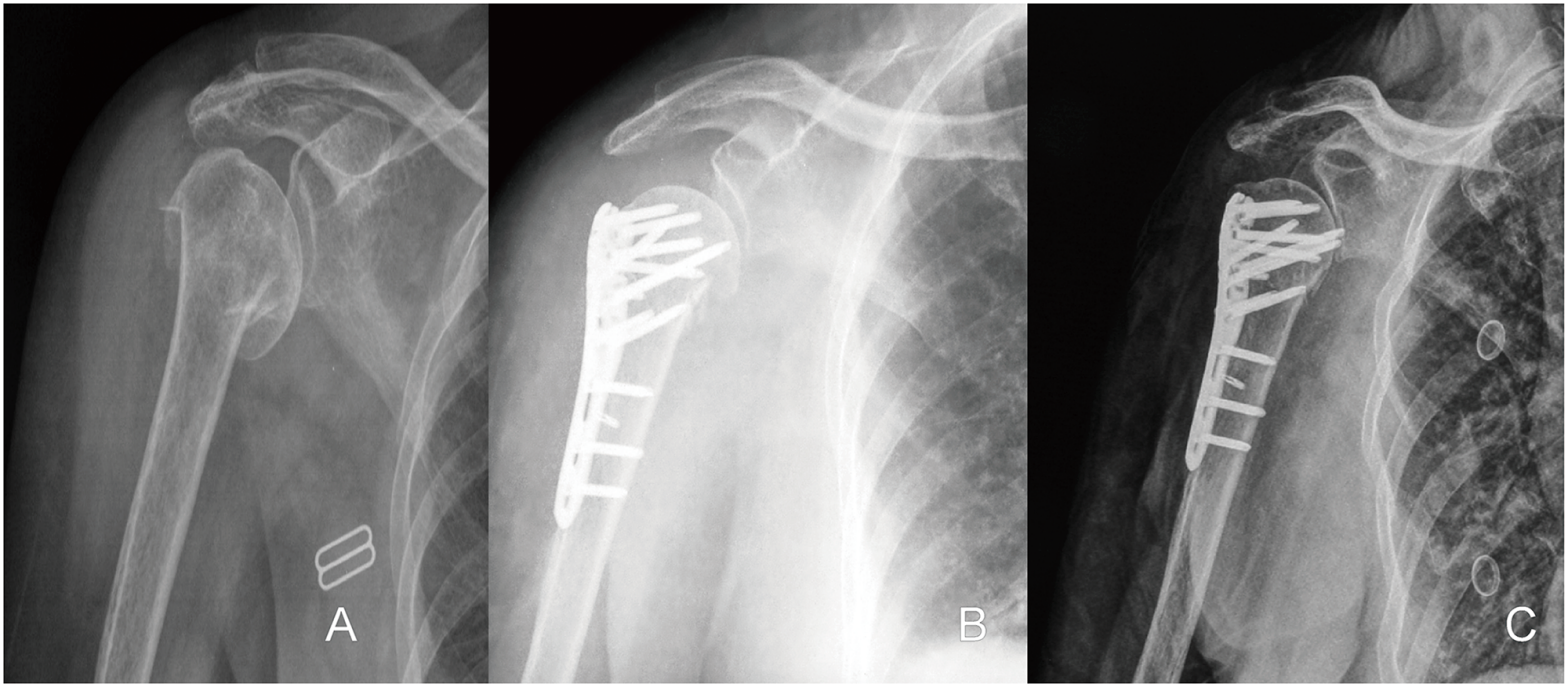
A: Anteroposterior shoulder radiograph of an 81-year-old woman who suffered a car accident in which her right shoulder impacted the ground, sustaining a 4-part (AO type C) proximal humeral fracture. B: the first day after surgery showing all screws within the humeral head. C: Two months postoperatively, screws penetrating the joint.
